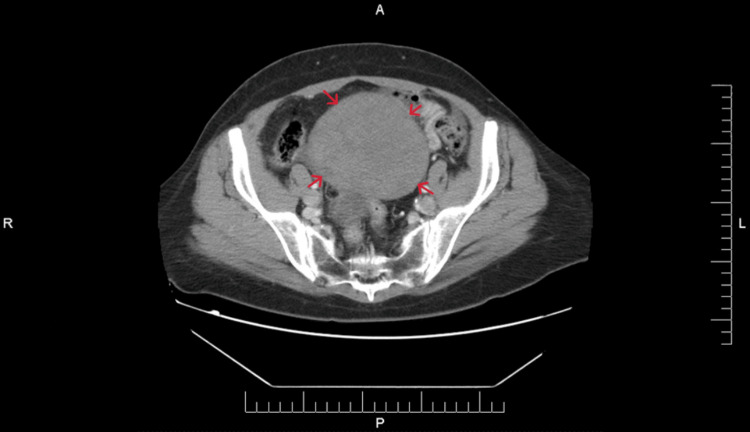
CT scan image of an ovarian fibroma with torsion A well-defined solid echogenic mass (red arrows) is clearly delineated in the pelvic cavity, most likely originating from the right ovary
